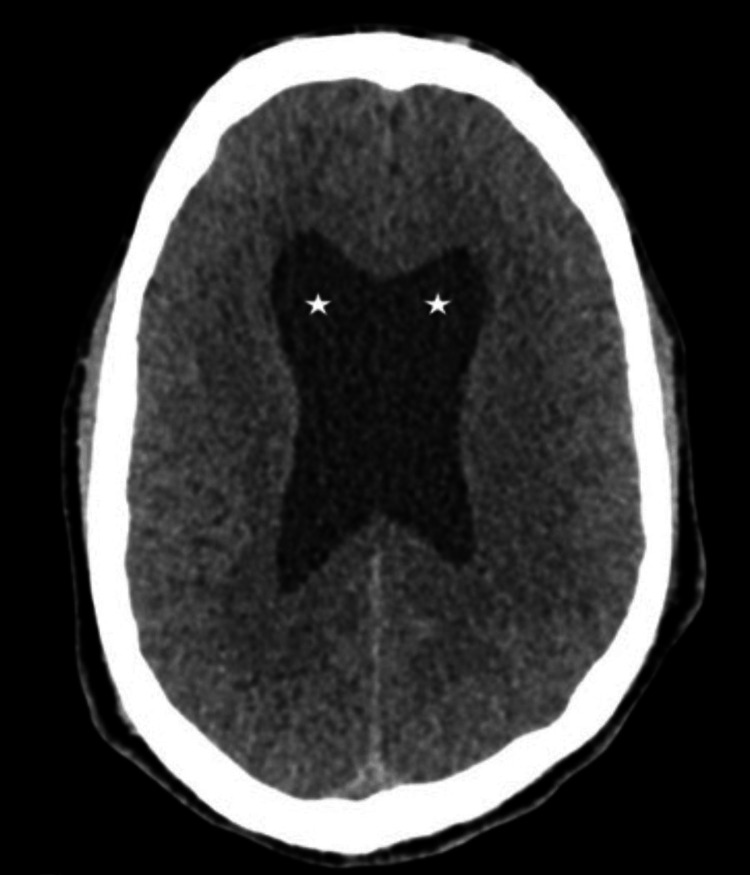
Brain CT showing dilated ventricular system with effacement of extra-axial cerebrospinal fluid (sulcal) spaces (white asterisks)
